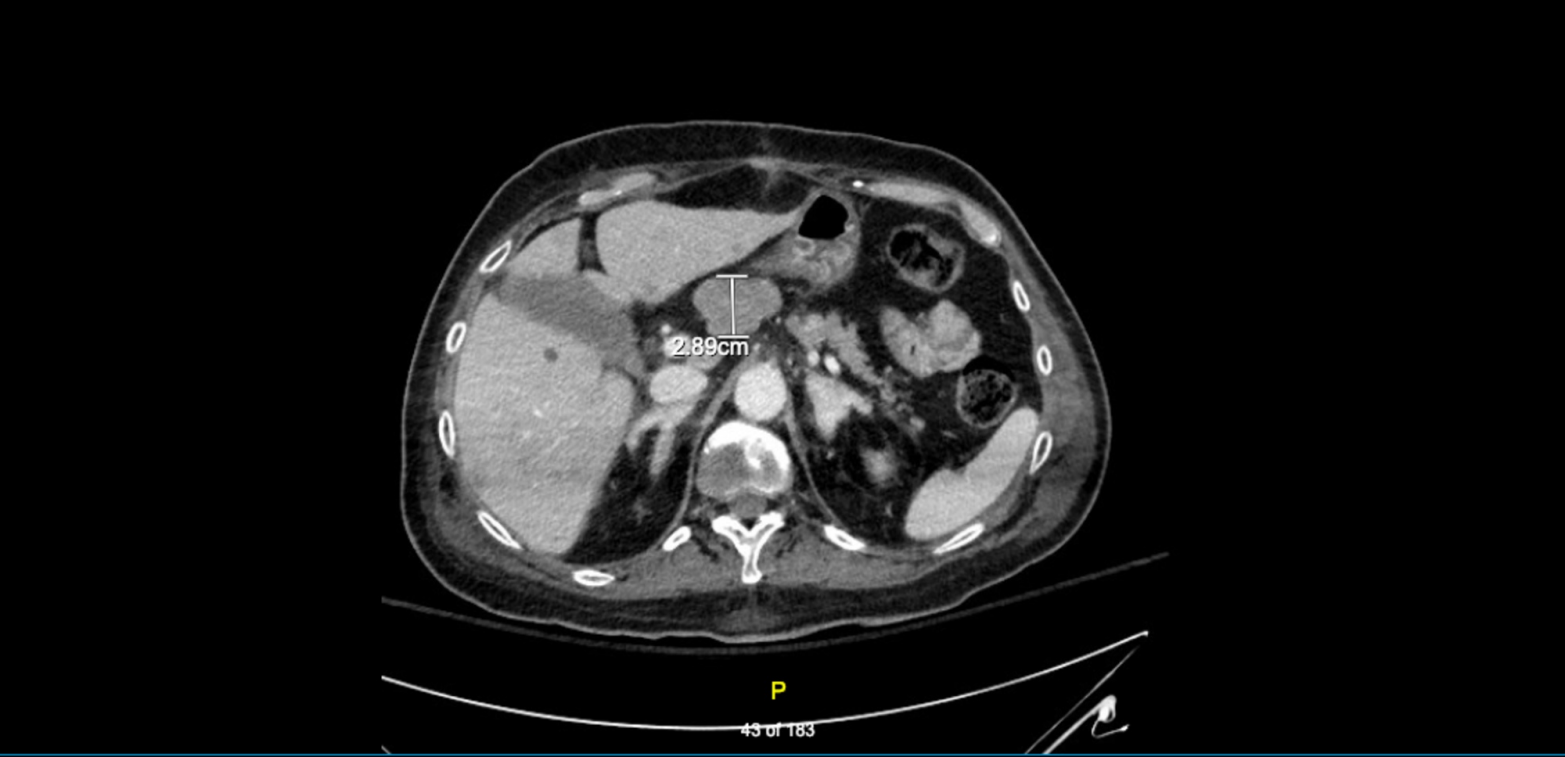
CT scan of abdomen with intravenous contrast. There is an ill-defined hypo-enhancing mass in the superior aspect of the pancreatic head which measures approximately 3.2 cm x 2.9 cm.
